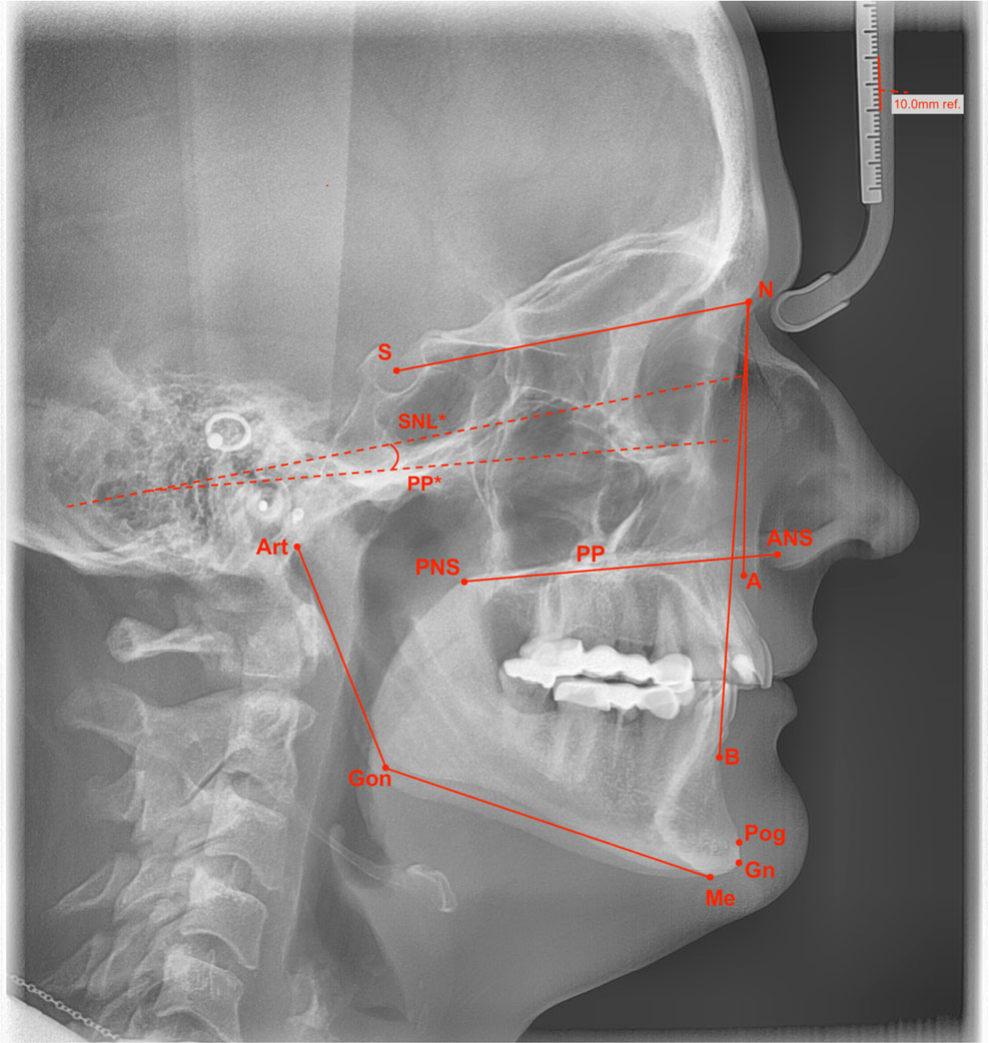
Lateral cephalometric radiograph with landmarks, lines and angles (red) serving as reference points. Landmarks are N, nasion; S, sella; A, A point (most concave point of anterior maxilla); B, B point (most concave point on mandibular symphysis); Pog, pogonion (most anterior point of mandibulae symphysis); Me, menton (lowest point on mandibular symphysis); Gn, gnathion (point of intersection between the line between pogonion and menton); Gon, gonion (point of intersection between ramus plane and mandibular plane); Art, articulare (intersection between the ascending ramus and the inferior surface of the cranial base); PNS, posterior nasal spine; ANS, anterior nasal spine, lines: SNL, sella-nasion line; PNS-ANS, maxillary length; Gn-Gon, mandibular plane; gonial angle, angle formed by a line connecting gnathion to gonion to articulare, and angles: SNA (°), SNA-angle (indicates whether or not the maxilla is normal, prognathic or retrognathic); SNB (°), SNB angle (indicates whether the mandible is normal, prognathic or retrognathic); ANB (°), ANB angle (indicates whether the relationship between maxilla and mandible is regular, a skeletal class II or class III. The patient has agreed in writing to the use of the X-ray image for publication.
